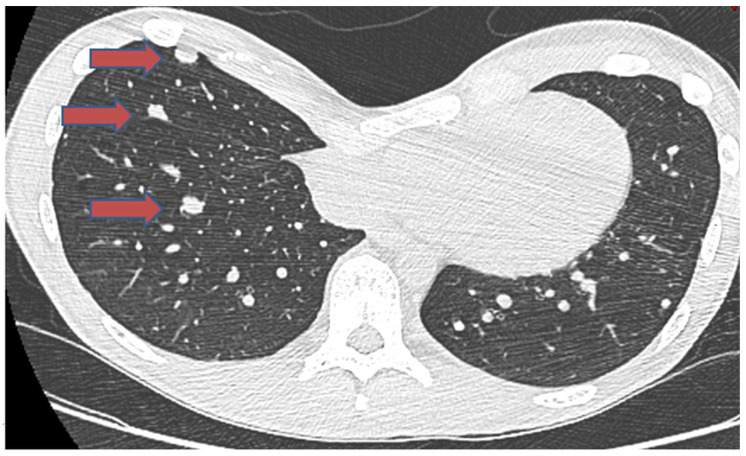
A chest CT scan, one year after denosumab treatment, showing a reduction in the size of 3 nodules and calcification marked by red arrows.
